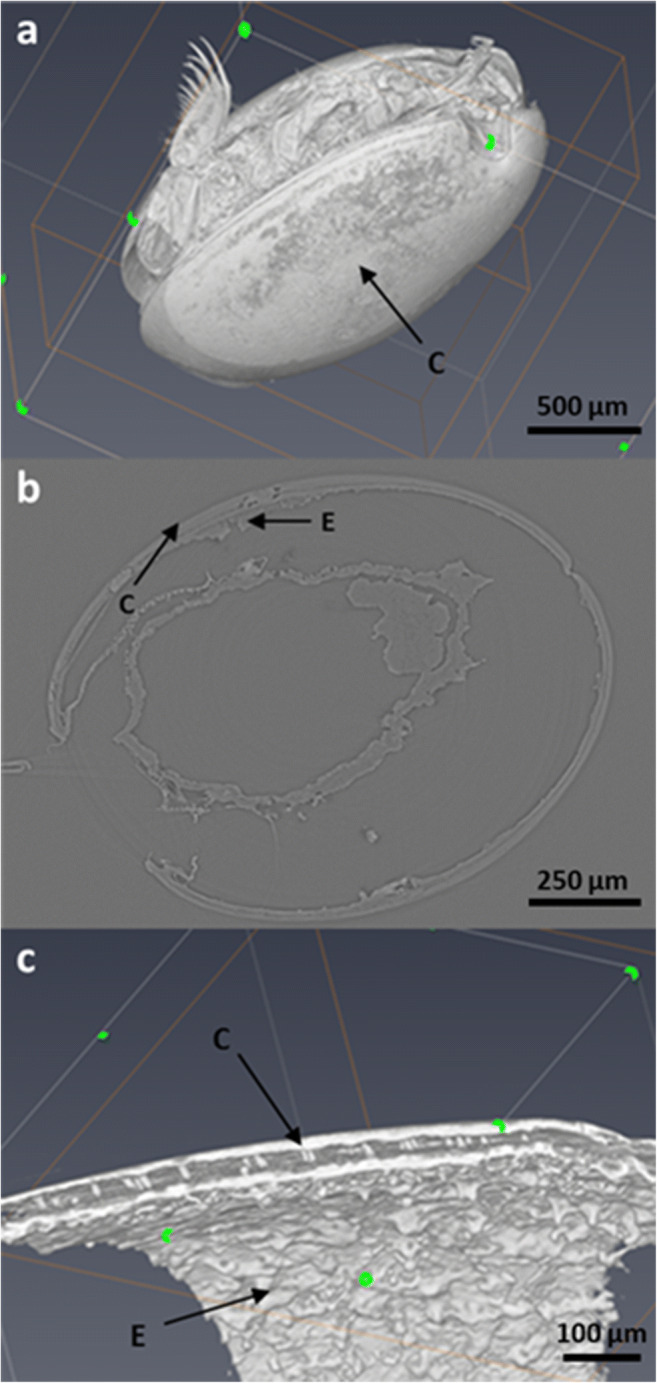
X-Ray microtomography reconstruction images of an adult ostracod used to understand ostracod carapace microstructure, prior to microdissection. (*a*) A whole adult ostracod rotated to reveal the inner body parts and appendages through the furca region of the partially open carapace. (*b*) Microtomography image slice at a depth of 810 μm from within a 3D image stack of an ostracod. (*c*) A cross-section of the ostracod bivalve displaying the layering of the carapace. *C* denotes ostracod carapace and *E* denotes underlying epidermal cell layer.
